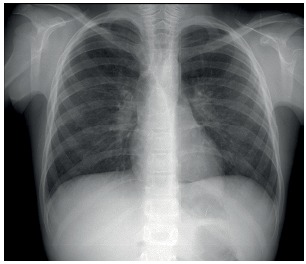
Chest X-ray showing bilateral perihilar and basilar reticulonodular
